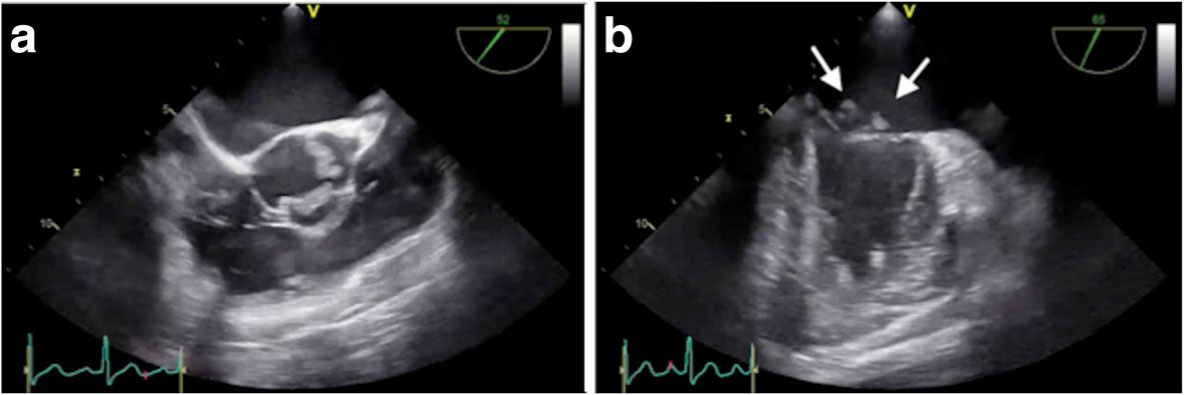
a Transesophageal echocardiogram shows structural change of left and right coronary cusps of aortic valve. b Vegetations on the mitral valve (*arrow*) in mitral commissural view. *LA* left atrium, *LV* left ventricle, *MV* mitral valve, *RA* right atrium, *TV* tricuspid valve, *RVOT* right ventricular outflow tract, *RV* right ventricle, *PV* pulmonary valve, *R* right coronary cusp, *L* left coronary cusp, *N* noncoronary cusp
